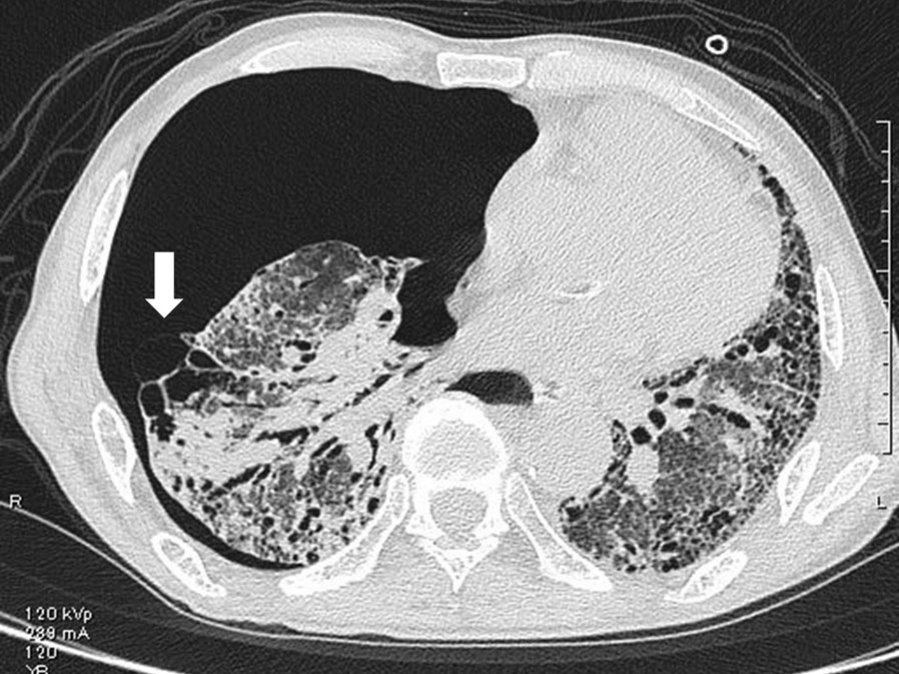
Computed tomography at the onset of pneumothorax showing cystic lesions in the fissure between the right middle and lower lobes (arrow)
